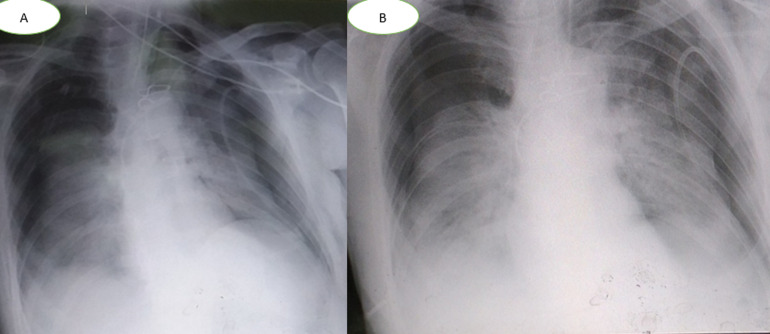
a chest X-ray demonstrating a return to the lung wall on the left side and the persistence of pneumothorax on the right side (A) and control after re-drainage (B)
